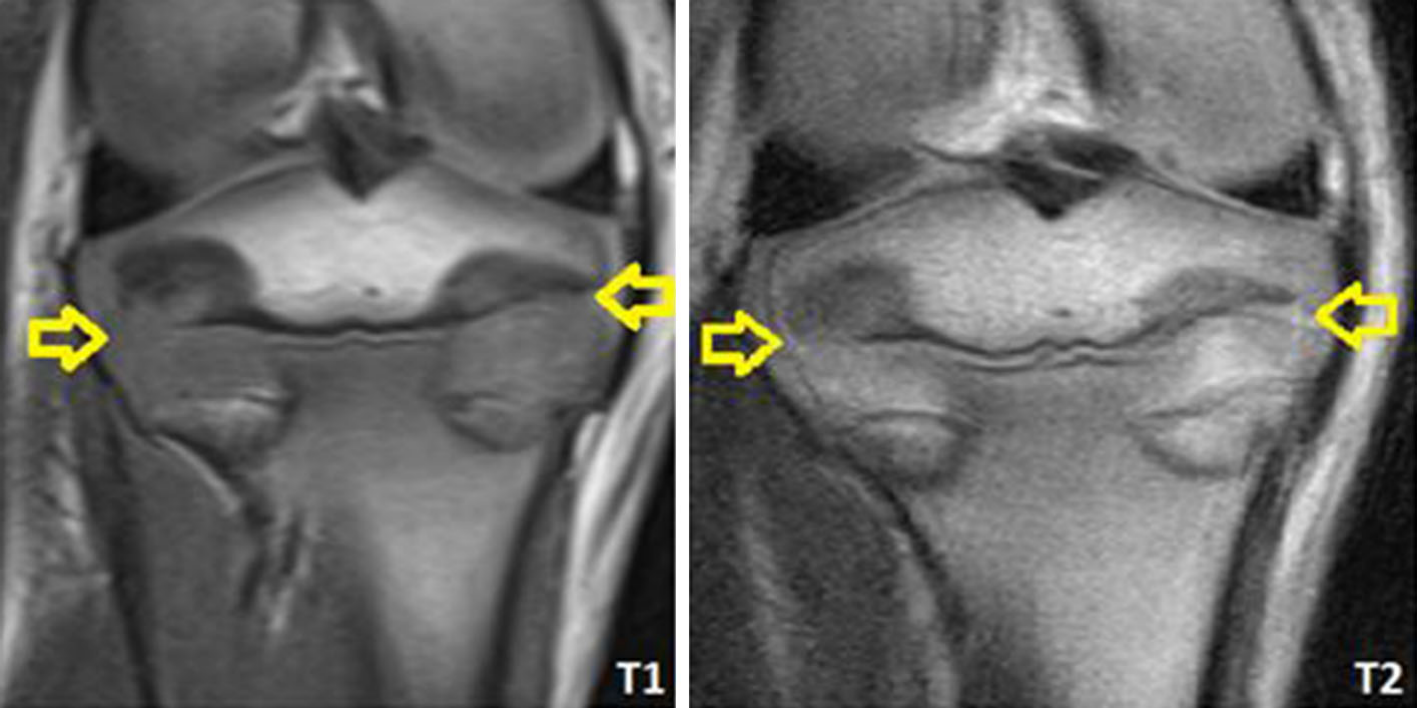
Magnetic resonance images (T1 and T2), study A. After the procedure, the physis can be observed to be disrupted (*arrows*). The articular cartilage above the epiphysiodesis looks continuous and homogenous
